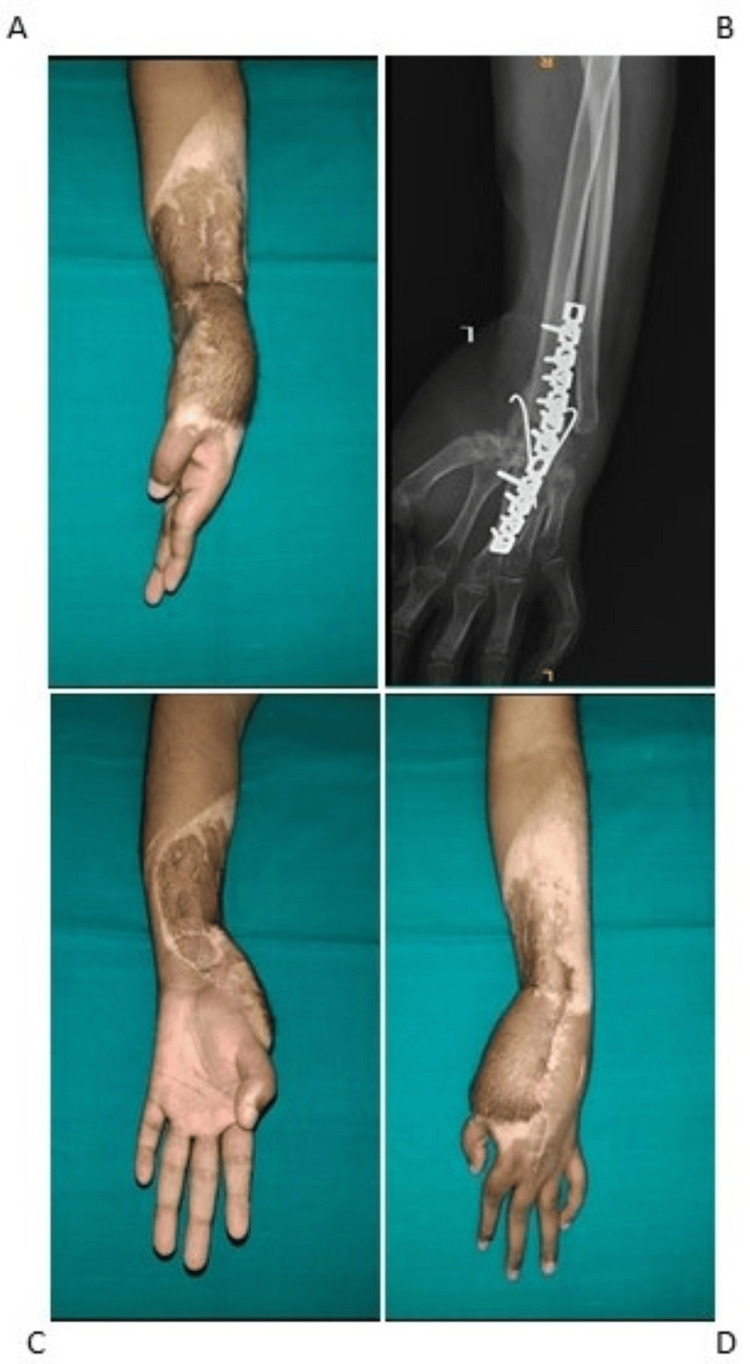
Postoperative correction of wrist flexion deformity of patient 1 (A). Postoperative X-ray of the right wrist after carpectomy and wrist arthrodesis of patient 1 (B). Reduction of deformity post-surgery on supination (C). Reduction of deformity post-surgery on pronation (D).
